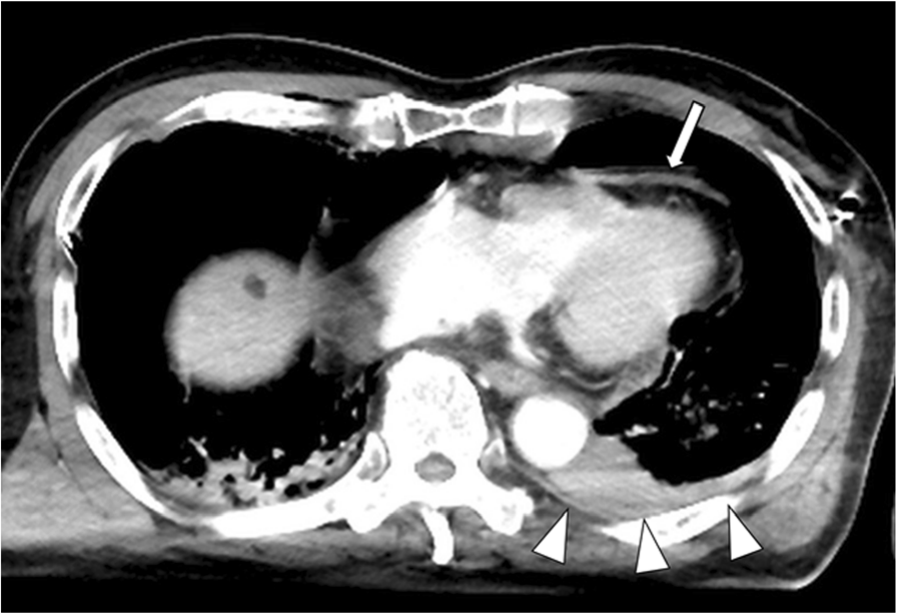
Initial chest computed tomography. Computed tomogram showed slight pericardial effusion (*arrow*). Computed tomogram showed left hemothorax with extravasation of contrast (*arrow heads*)
